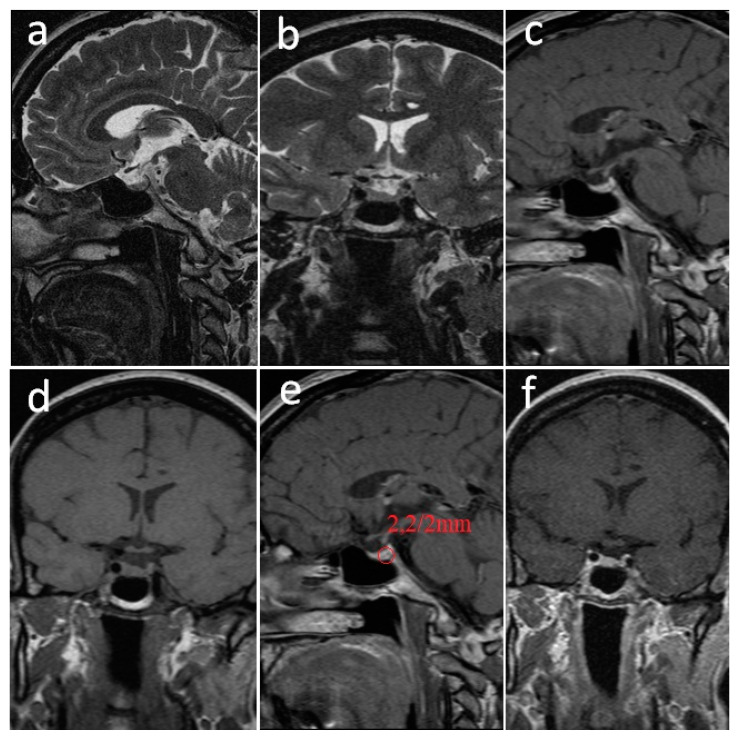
Pituitary MRI performed 6 months after delivery. (a). Non-contrast sagittal T2-weighted image. (b). Non-contrast coronal T2-weighted image. (c). Non-contrast sagittal T-weighted image. (d). Non-contrast coronal T1-weighted image. (e). Post-contrast sagittal T1-weighted image. (f). Post-contrast coronal T1-weighted image. The imaging aspect was the same as that at the beginning of the pregnancy; the pituitary adenoma was located in the left parapituitary in moderate high-signal T2-weighted (a,b), low-signal T1-weighted (c,d), and non-gadolinium enhanced (e,f). The diameter of the pituitary adenoma was 2/2 mm.
